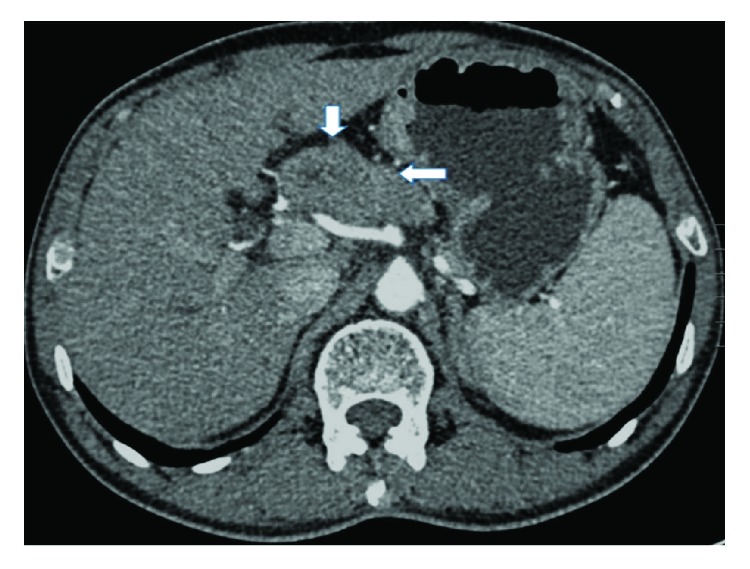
CT image obtained in a 64-year-old man. A big lymph node with a short-axis diameter > 8 mm can be seen along the lesser curvature of the stomach.
